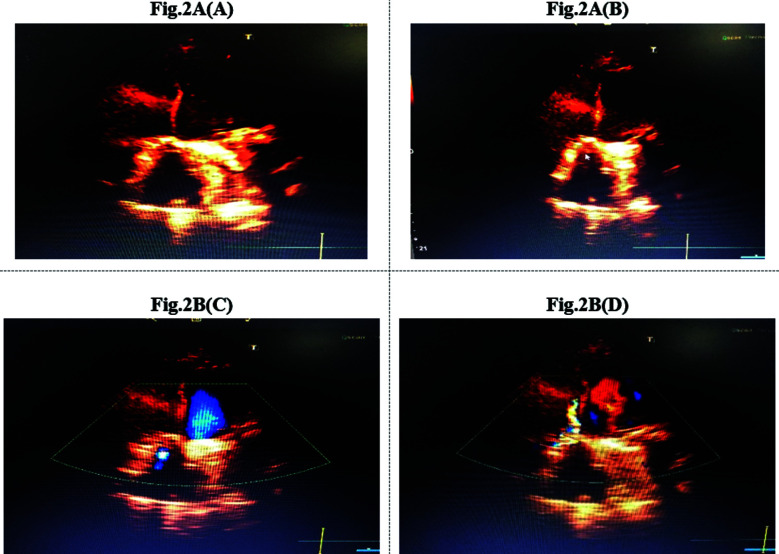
TTE of the mechanical tricuspid valve prosthesis. (A), Systolic and (B) diastolic frames show that both discs are stuck in an open position (arrows). (C), Color Doppler showing a mobile structure is intermittently noted in the medial inflow portion of the prosthesis suggestive of a Pannus/thrombus formation. (D), a turbulent diastolic jet across the prosthesis.
